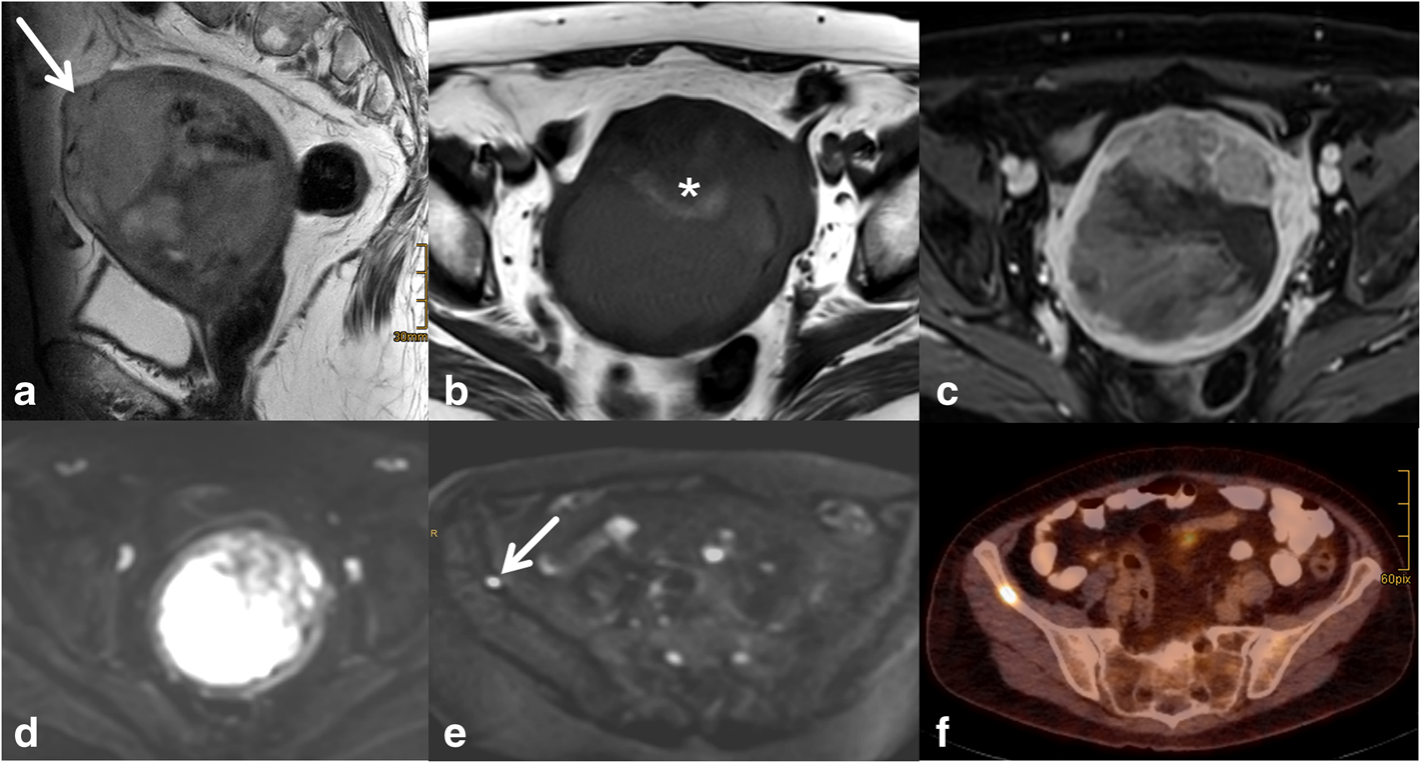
Subserous tumour spread. Type II endometrial cancer (grade III) with subserous uterine growth (a, arrow). Transaxial T1WI demonstrates hemogeneous structure with hemorrhage (b,*). Necrosis is seen on T1GD FS (c). Transaxial DWI (d) at the level of the primary tumour and above (e) demonstrate high signal on b 1000. The focal osseous lesion with high signal on high b value image corresponds to a bone metastasis in PET/CT (f)
